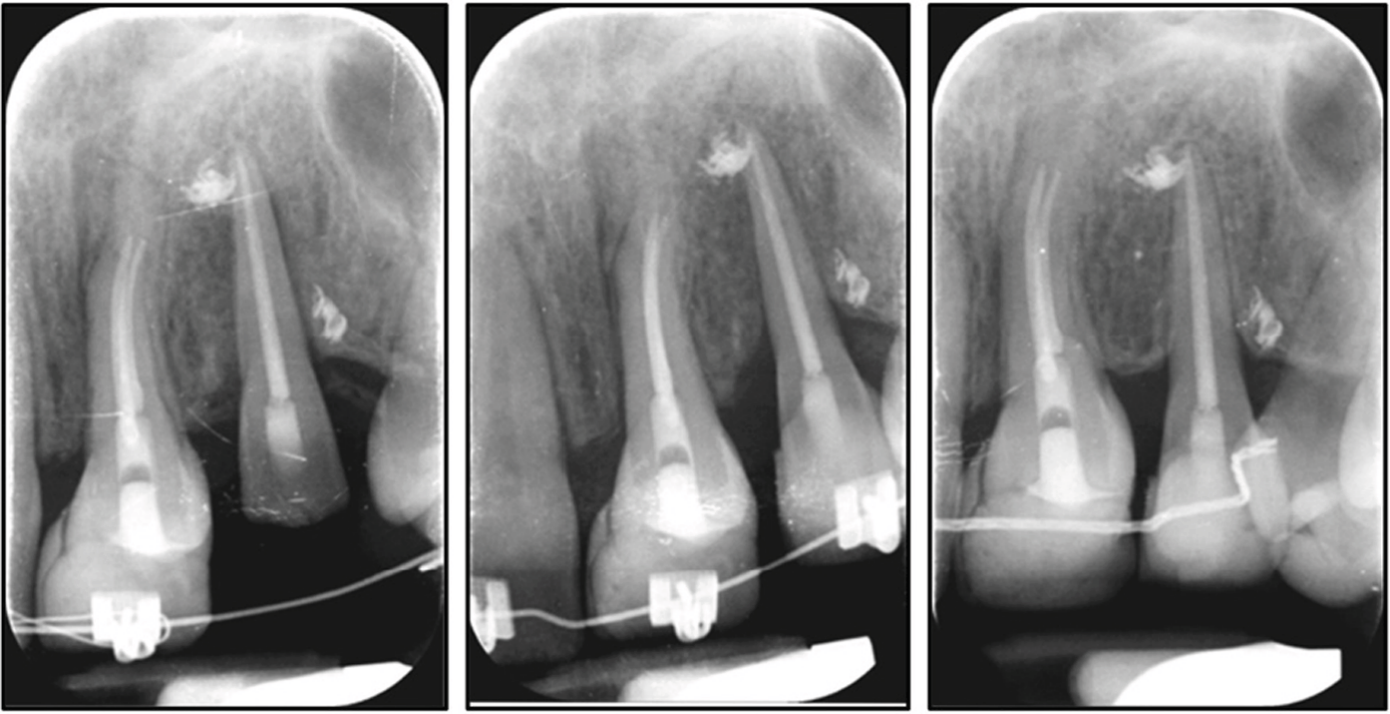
Radiographic evaluation of Tooth 3.1 during extrusion and after its transplantation and bonding.
